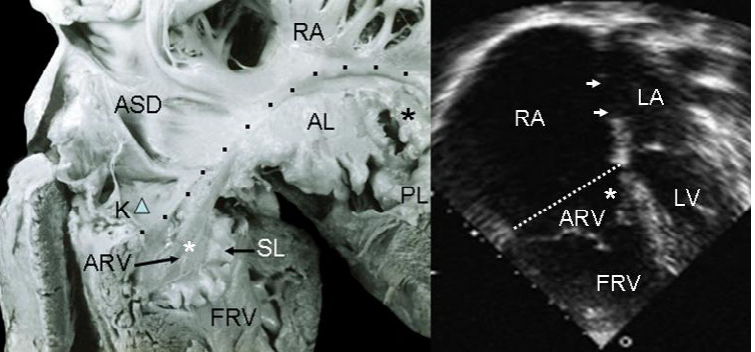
Internal view of the right chambers shows mild (Grade I) tethering of the tricuspid septal leaflet (asterisk). Note the nodular dysplasia of the free portion of the leaflet. Koch's triangle over the atrioventriculaar septum and the atrial septal defect are visible. The 4 chamber echocardiographic image shows grade I tethering of the septal leaflet. The atrialized portion of the right ventricle is smaller than the functional portion. The double arrow points to the atrial septal defect. K: Koch's triangle. Other abbreviations as before.
